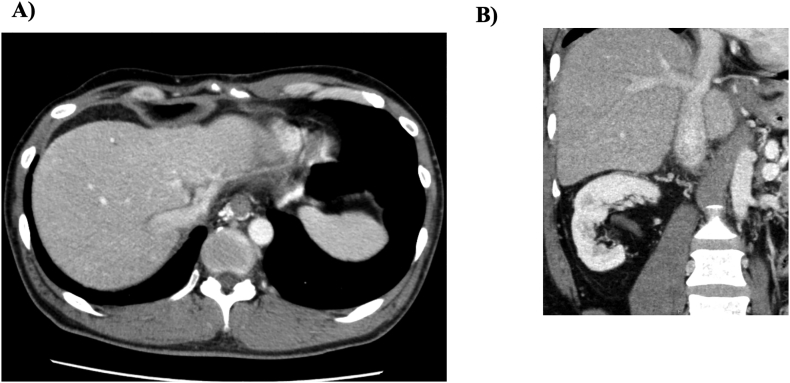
Postoperative contrast-enhanced computed tomography. A) Wide orifice of the right and left hepatic vein. Homogenous enhancement of the liver parenchyma. B) Improvement of the stenotic lesion in the IVC.
